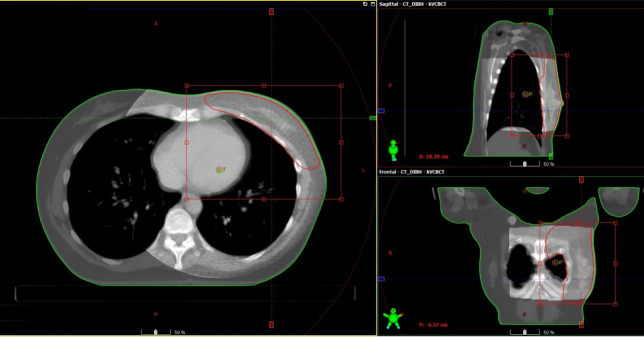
Align the CBCT with the positioning CT images.
